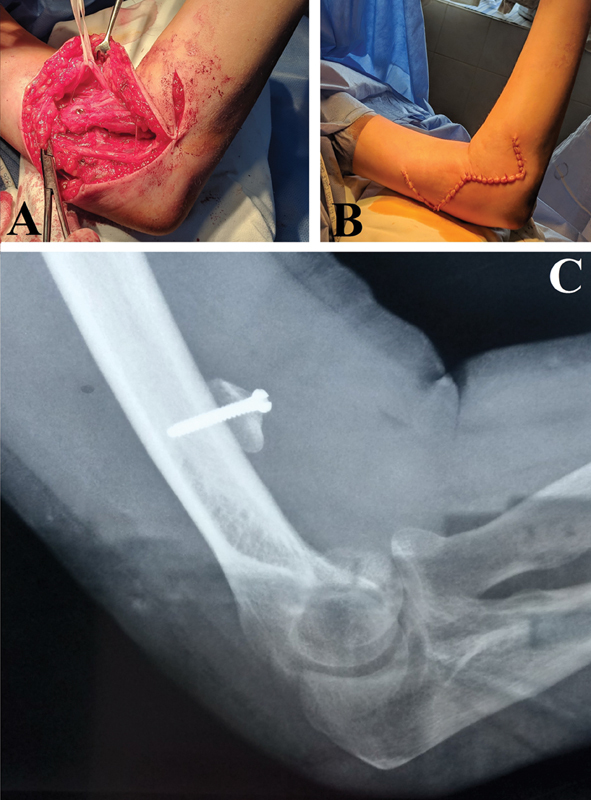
Intraoperative and X-ray modifications of Steindler flexorplasty by Loeffler and Lewis
